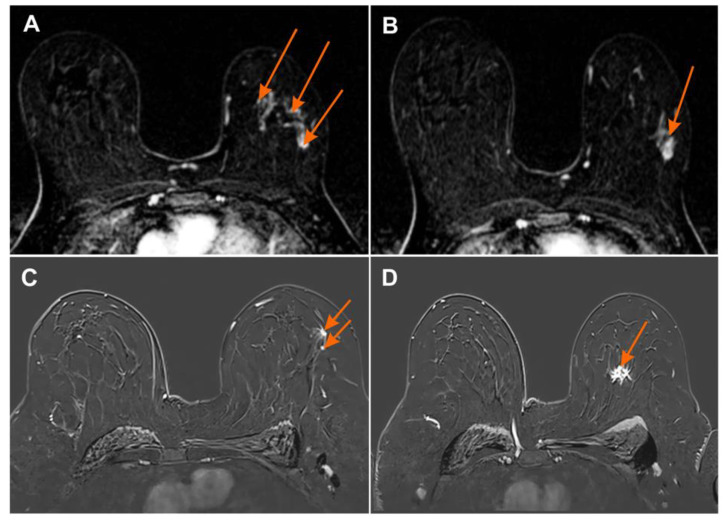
Magnetic resonance imaging (MRI) subtraction image (A,B) 2 min after contrast injection irregular mass with heterogeneous enhancement BI-RADS 6 with numerous foci of contrast uptake showing multicentric cancer BI-RADS 4 (orange arrows); (C,D) MRI subtraction image 2 min after contrast injection irregular mass with heterogeneous enhancement BI-RADS 6 with additional foci of contrast uptake showing multicentric cancer BI-RADS 4 (orange arrows).
